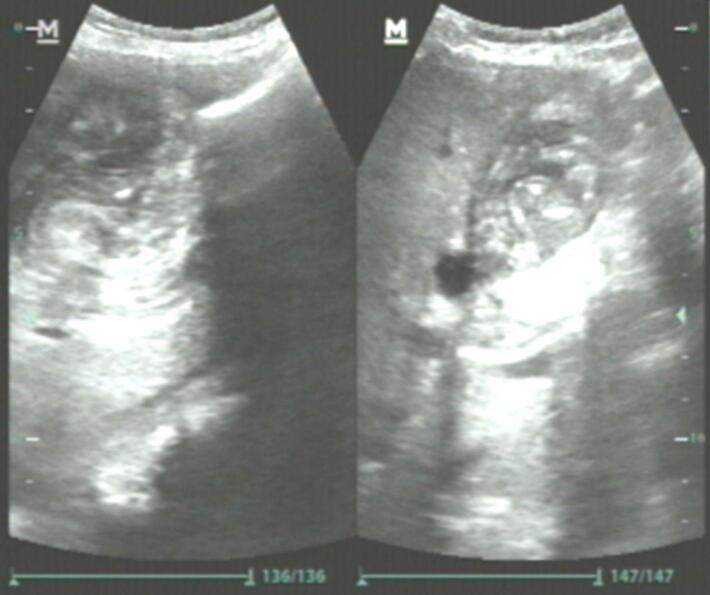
Ultrasound showing multiple linear echogenic structures in gallbladder.
